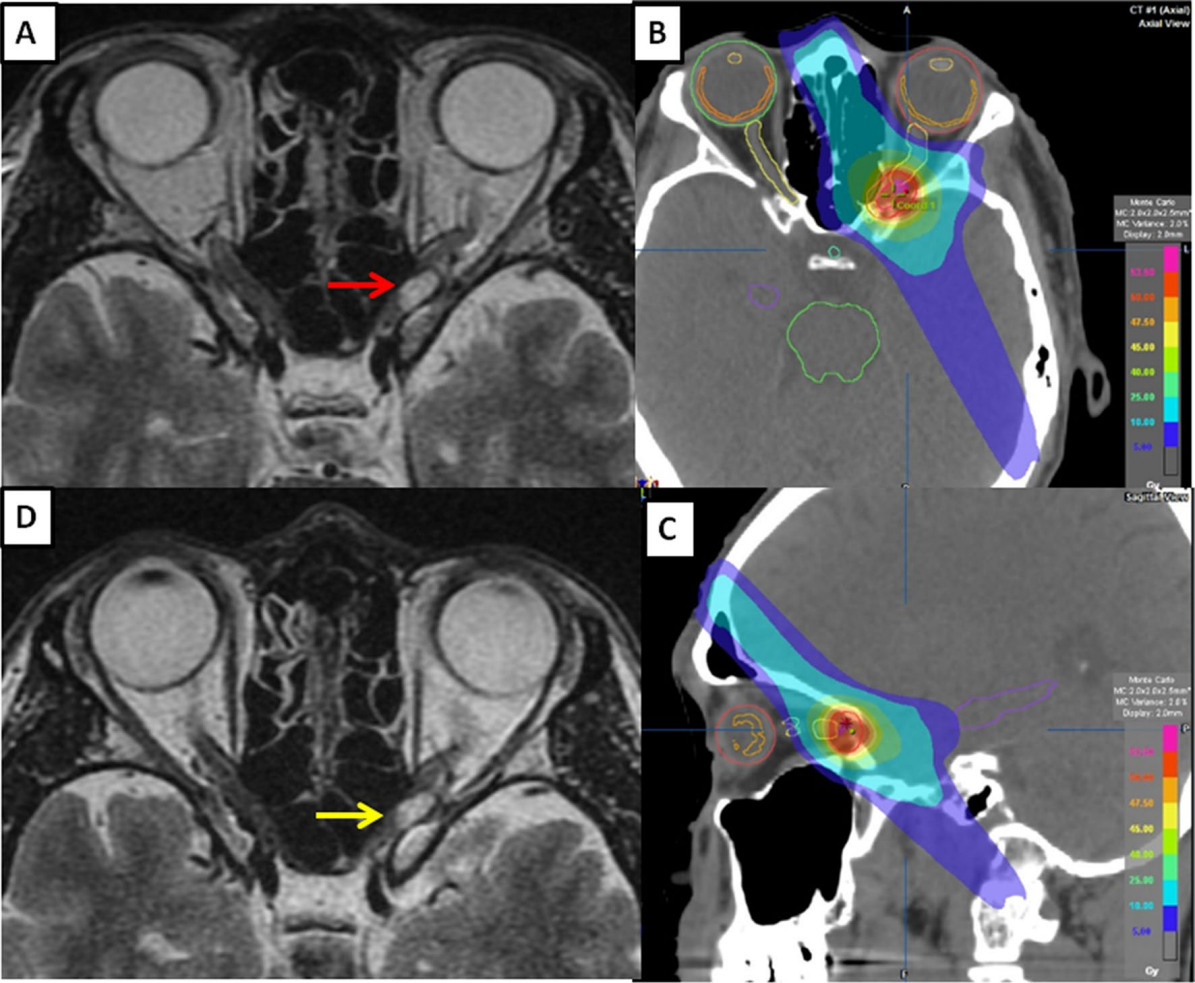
Pretreatment magnetic resonance imaging (MRI) showed a fusiform type of intraorbital tumor (red arrow) at the pre-canalicular portion of the left optic nerve (a). The patient underwent intensity-modulated radiotherapy (IMRT) at a dose of 50 Gy delivered in 25 fractions over 35 days in May 2018 (b, c). Despite a rapid improvement in visual field, detected with Humphrey automated perimetry, MRI did not show any significant change in tumor size (yellow arrow) during the early stages of IMRT (d)
